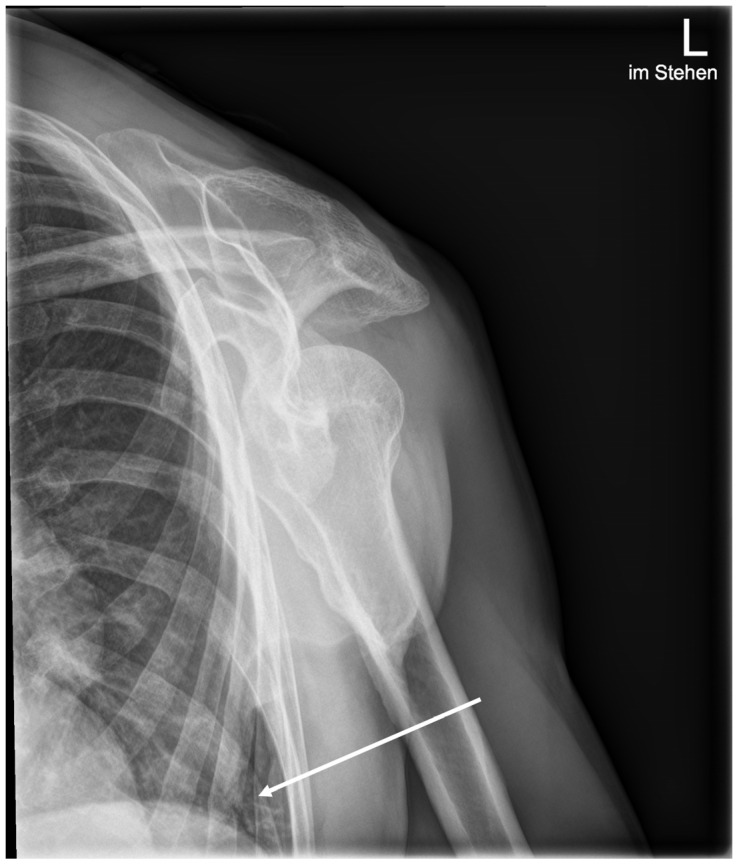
Standing ap radiograph of the left shoulder. The white arrow marks an incidentally caught rib fracture, which was correctly diagnosed by the resident but missed by the AI software.
